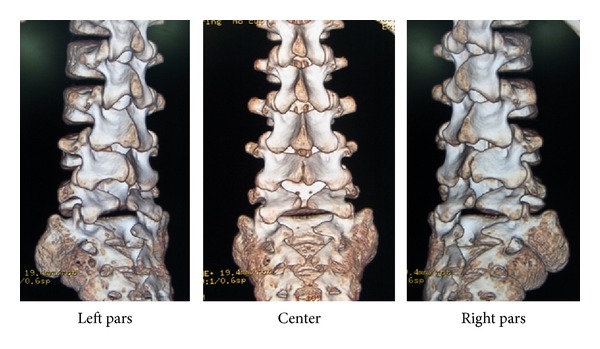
3D-CT of Case 2. Note the concomitant existence of the right side L5 unilateral spondylolysis and SBO.
